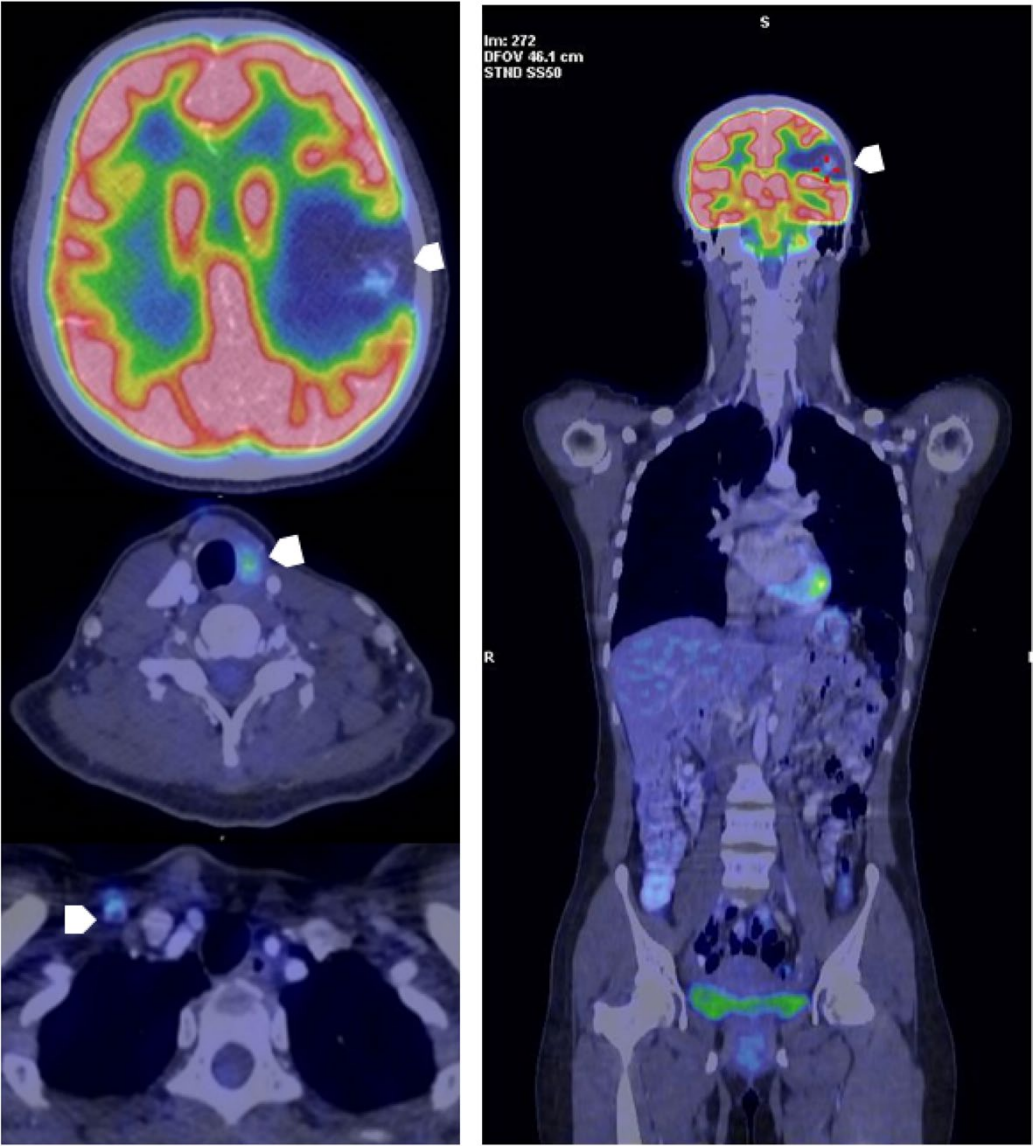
^18^F- FDG PET/CT images A decreased.^18^F-FDG avid metastatic lesion in the left temporal lobe and the thyroid bed recurrent lesions and the right supraclavicular lymph nodes metastases (arrow head)
